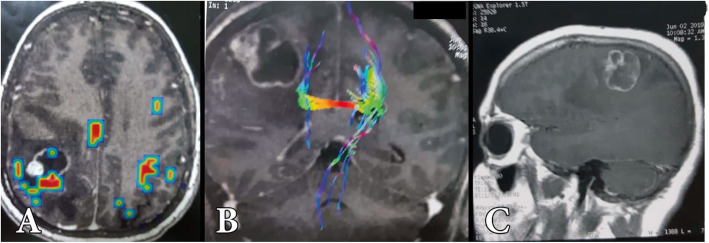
Preoperative axial bold (A), coronal tractography (B), and sagittal post-contrast MRI images of a 32-year-old female patient presented by seizures and hemiparesis grade 4 history of cancer ovary with oopherectomy and hysterectomy since 2 years
